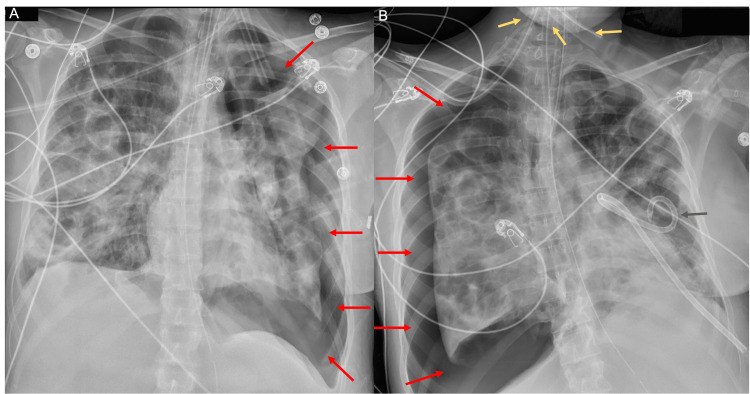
Portable semi-erect anterioposterior radiograph of the chest demonstrates a left pneumothorax (delineated by red arrows in A) without mediastinal shift. A subsequent radiograph of the chest demonstrates a large right pneumothorax (delineated by red arrows in B) with a possible right-to-left mediastinal shift. Thin, linear, metallic foreign bodies are noted involving the base of the neck (yellow arrows in B). A left-sided chest tube is present (dark gray arrow in B).
